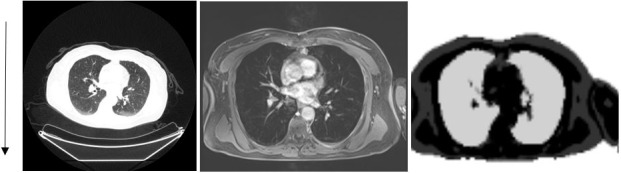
Example computed tomography (CT) [Left], magnetic resonance (MR) [Middle], and MR attenuation correction (MRAC) [Right] images of the lungs. An increase in CT intensity can be seen in the direction of gravity (arrow) but this change in density is not reflected in the MRAC.
